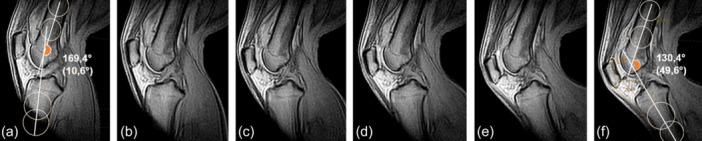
Representative image series of dynamic magnetic resonance imaging (MRI) of a knee from near extension to increasing flexion (a: 10°; b: 17°; c: 25°, d: 30°, e: 38°; f: 49° of flexion).
